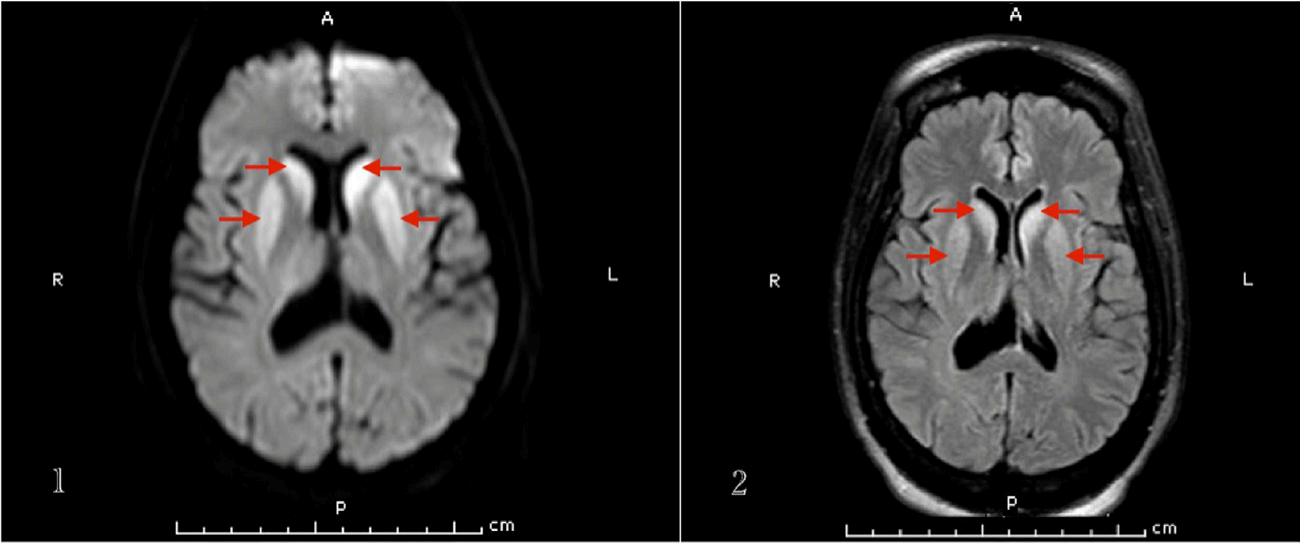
MRI brain (1. DWI, 2. FLAIR sequence) of patient 3 that demonstrates basal ganglia hyperintense signal.
